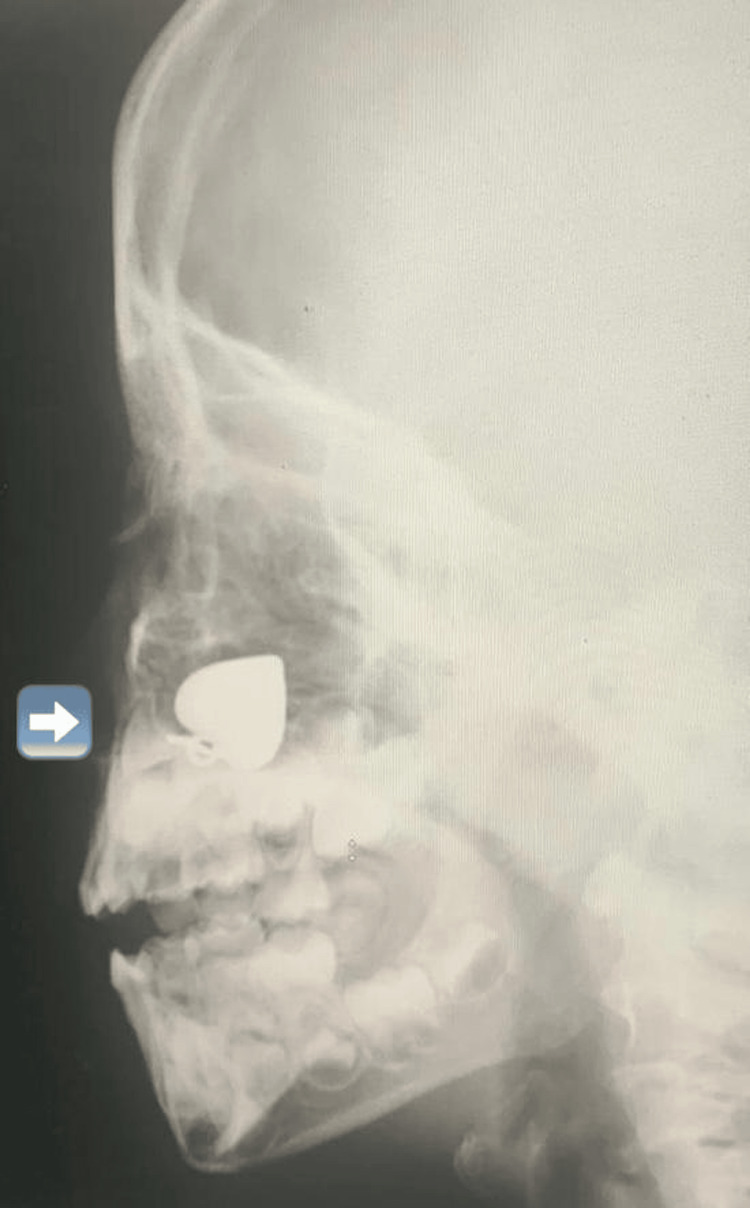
X-ray showing a metallic spade-shaped foreign body in the nasal cavity
